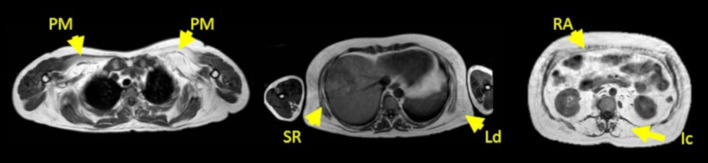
MRI T1w of patient 14 at first visit. From left to right, first image corresponds to shoulder girdle level; second image corresponds to a thoracic level, and third image corresponds to an abdominal level. Yellow arrows point to the pectoralis major (PM), serratus (SR), latissimus dorsi (Ld), rectus abdominis (RA), and iliocostalis muscle (Ic). The MRI scores at this visit were 30 points in abdominal score, 25 points in paraspinal score, and 18 points in thoracic score. The Global score was of 73 points.
